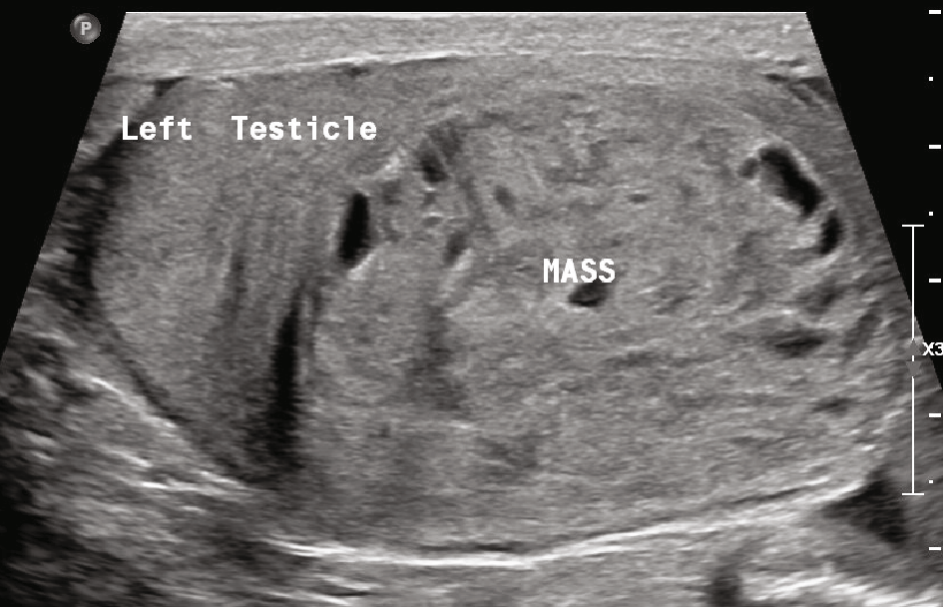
B-mode ultrasound reveals a well-circumscribed intratesticular mass lesion involving the left testicular parenchyma in the inferior aspect. The lesion is heterogeneous and predominantly solid with several small cystic spaces.
